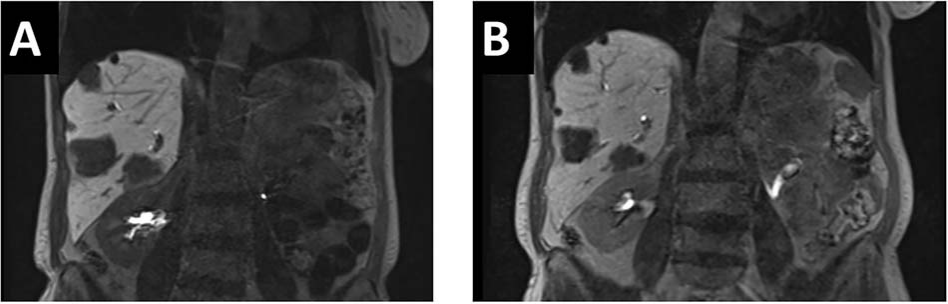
(A). In the initial pre-chemo MRI of the liver multiple liver metastases in all liver segments could been seen. The largest were in segments II/III (26 mm), V (37 mm) and segment VI (40 mm). (B) After 12 cycles of chemotherapy a size reduction of liver metastasis up to 12% could be achieved. The metastases in liver segments V and VI decreased from 43 to 38 mm and from 37 to 33 mm.
